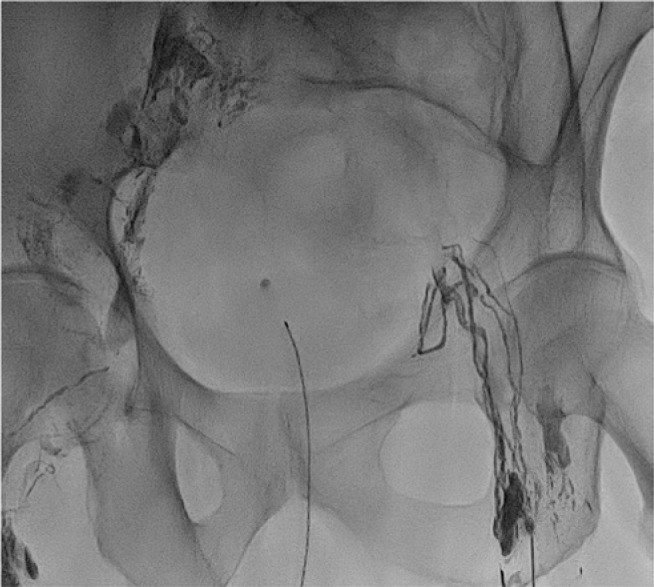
Bilateral groin lymphangiogram: after ultrasound-guided bilateral lymph node puncture, Lipiodol is infused into the lymphatics. The spot radiograph shows the initial phase with opacification of inguinal lymph nodes and lymphatic drainage of the pelvis.
